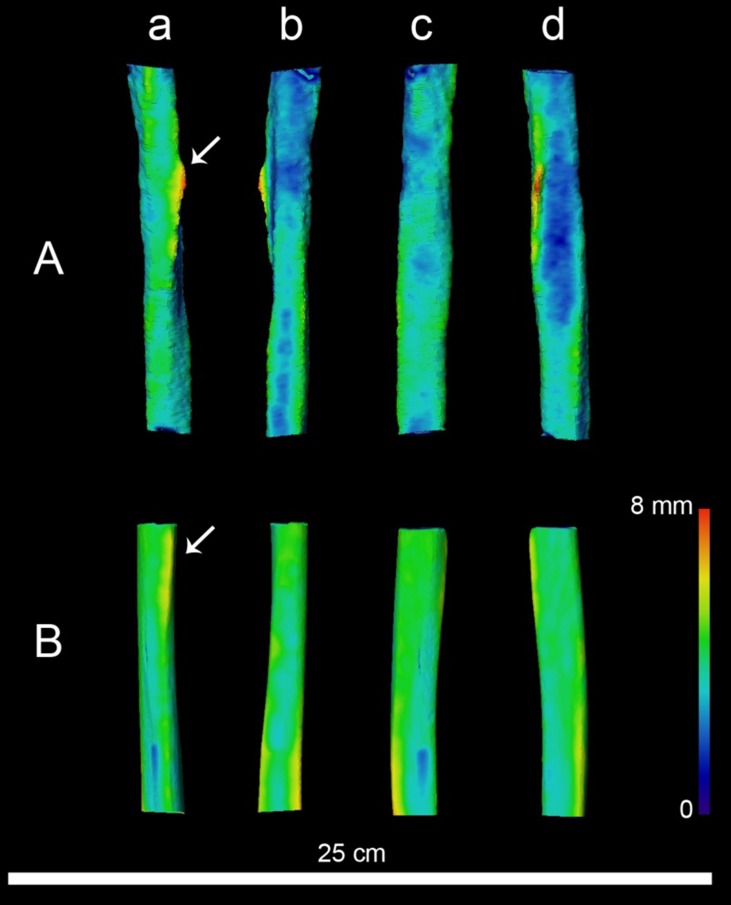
CT-based 3D mapping of the topographic distribution of the cortical bone at the proximal part of the humeral diaphysis (A) compared to the equivalent area (B, 6 cm below the deltoid tuberosity) on the humerus of the Krapina # 165 Pre-Neandertal (NESPOS data base, [40]) (B). Cortical thickness topographic variation was rendered using a chromatic scale increasing from dark blue (thin) to red (thick). The arrows indicate the position of the deltoid tuberosity in both shafts. a: anterior view, b: posterior view, c: medial view, d: lateral view.
